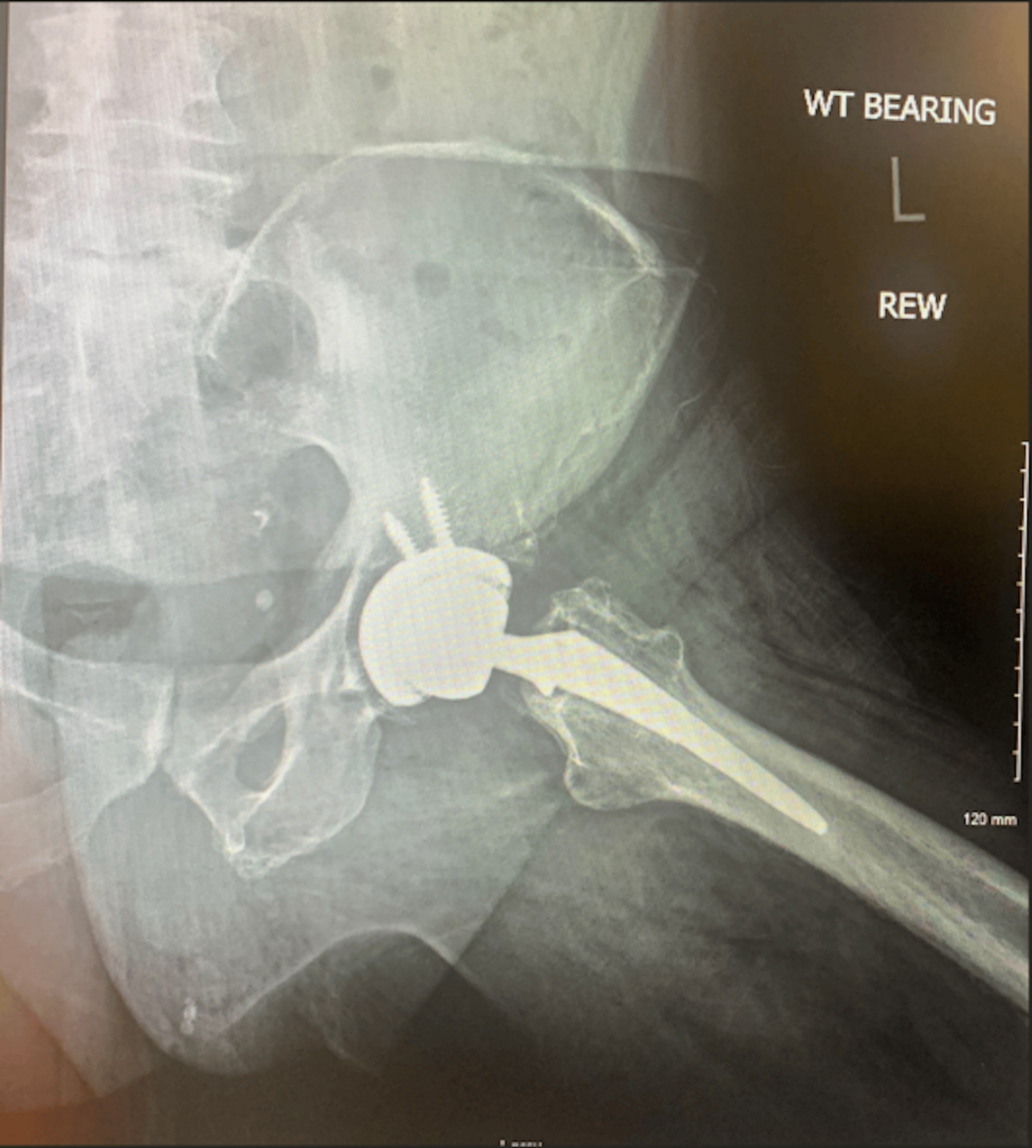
Postoperative Dunn view radiograph of the left hip
